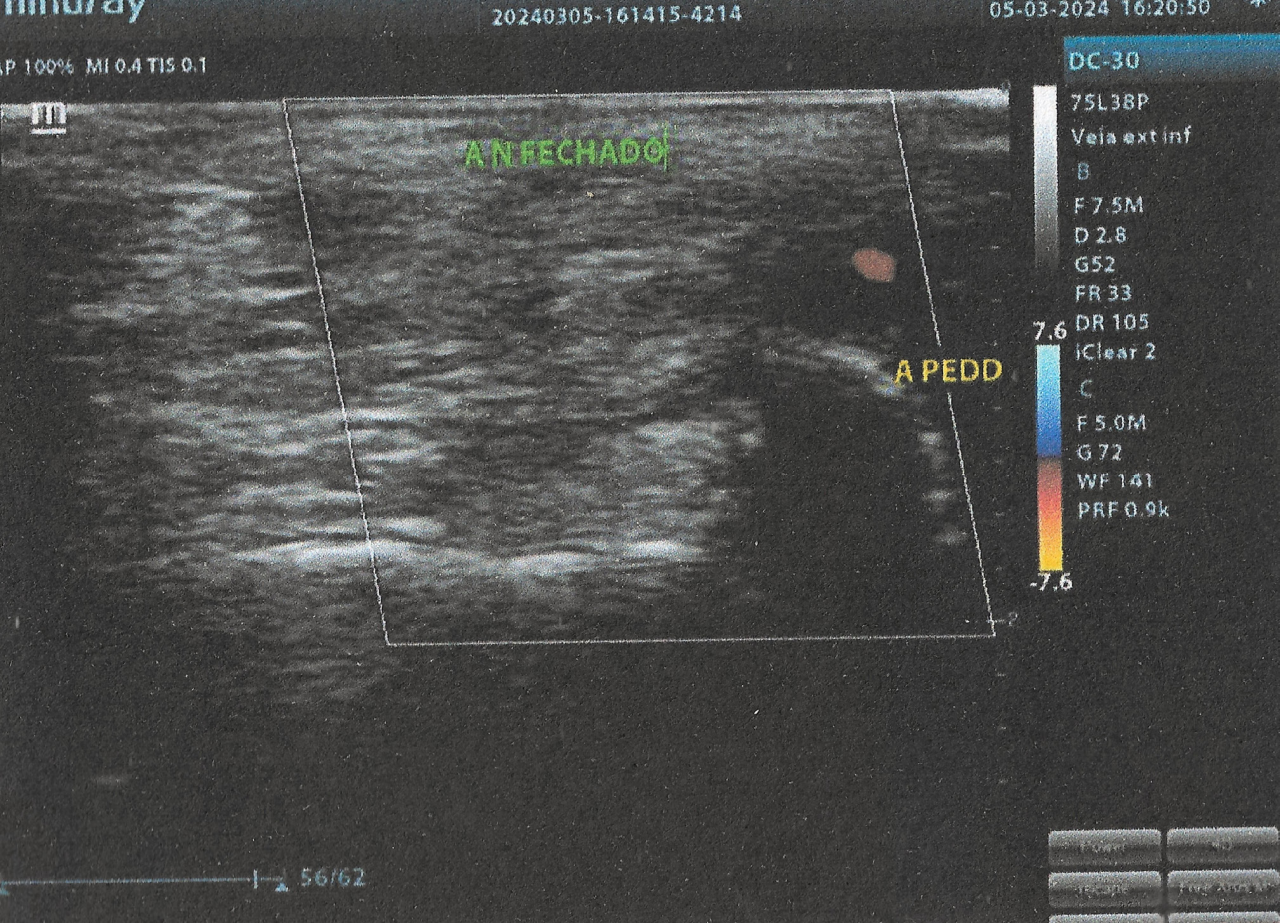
US with Doppler. Aneurysm of approximately 7 mm in size, without flow (CLOSED AN.) and dorsalis pedis artery (D. PED. A.) perfused by retrograde flow from the plantar arch.
